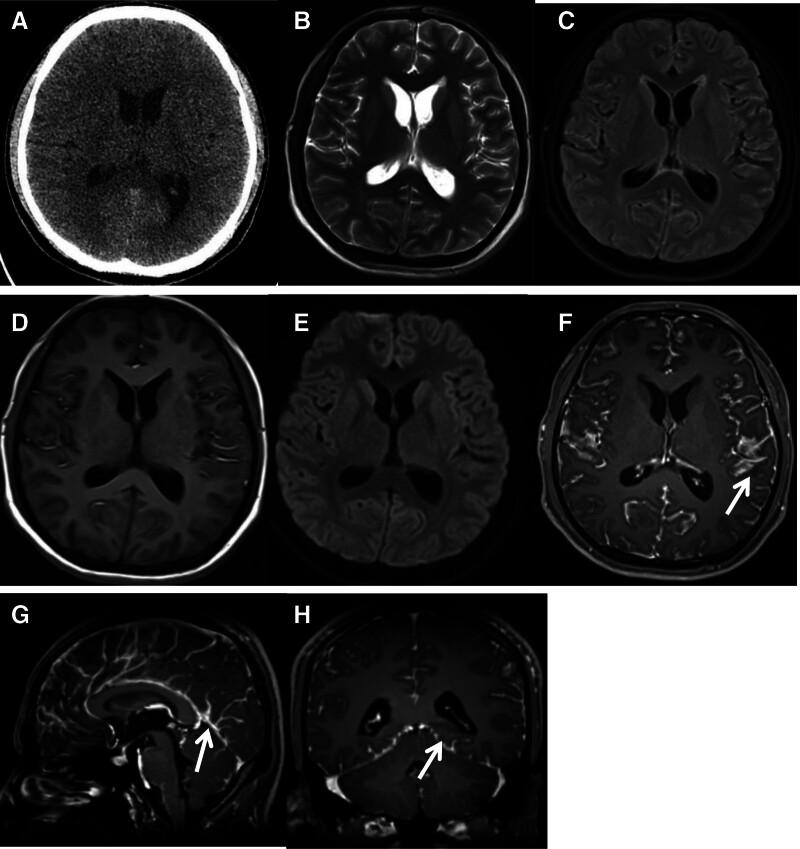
Radiologic findings. (A) Plain cranial CT: no abnormal density. (B–C) T2WI and FLAIR: subtle cortical hyperintensity. (D) T1WI: linear structures within the cerebral sulci of both hemispheres; definitive T1 shortening attributable to melanin was not conspicuous. (E) DWI: no restricted diffusion. (F–H) Post-contrast T1WI: diffuse linear and strip-like leptomeningeal enhancement (arrow). FLAIR = fluid-attenuated inversion recovery.
